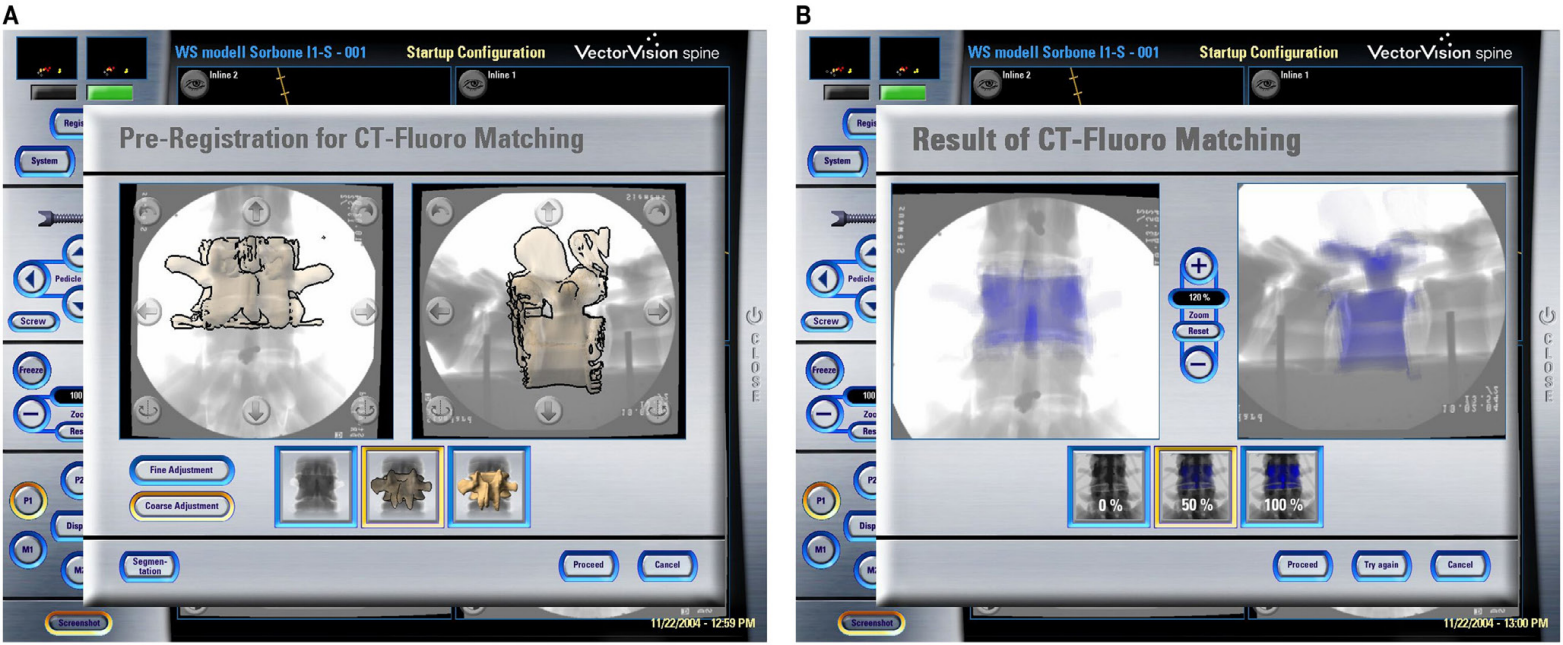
CT-Fluoro matching. Screenshots of different stages of a CT-Fluoro matching process. (A) Pre-registration for CT-Fluoro matching; and (B) results of CT-Fluoro matching (Courtesy of BrainLAB AG, Munich, Germany).
